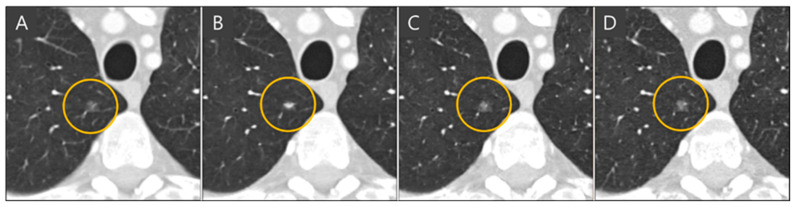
Axial view of (A) thick-slice, (B) generated thin-slice produced by the model trained on a single slice, (C) generated thin-slice produced by the model train on multiple slices, and (D) thin-slice CT images, subsolid nodules are circled.
